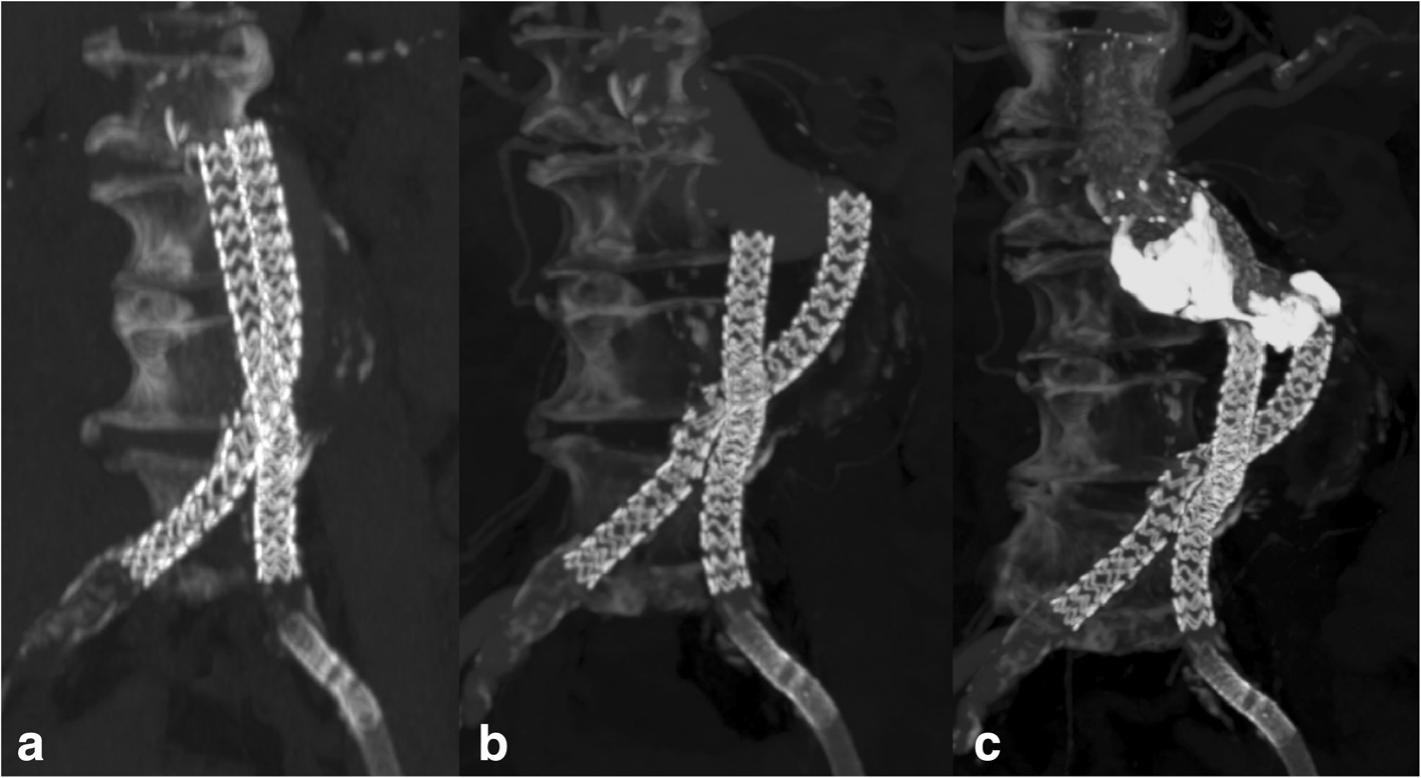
a) CT-Angio 24 months after stentgraft placement. The two graft limbs are intact and unchanged in position, conformation, and alignement. b) CT-Angio 36 months after stentgraft placement. The limbs have separated and fallen into the stomach-shaped aneurysm. Note the type Ia endoleak. c) CT-Angio 1 month after secondary intervention: 1. Extension of both limbs with stentgrafts until beneath the renal arteries 2. Deployment of additional bare stents above the renal arteries for further stabilization 3. Injection of liquiq embolics (Onyx) into the aneurysmatic sac to seal the type Ia endoleak. Renal arteries were normally perfused and the endoleak sealed after treatment
